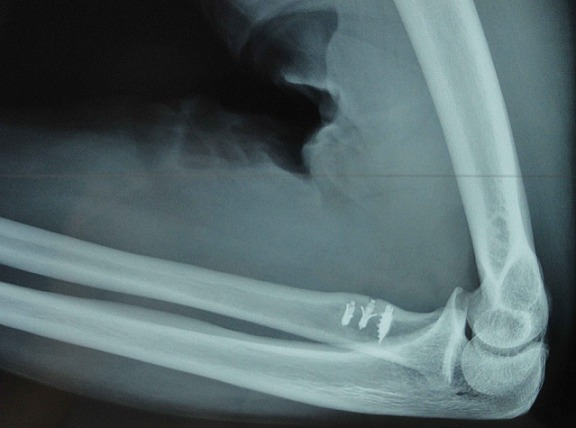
Lateral radiograph of the elbow showing trans-osseous anchors position
